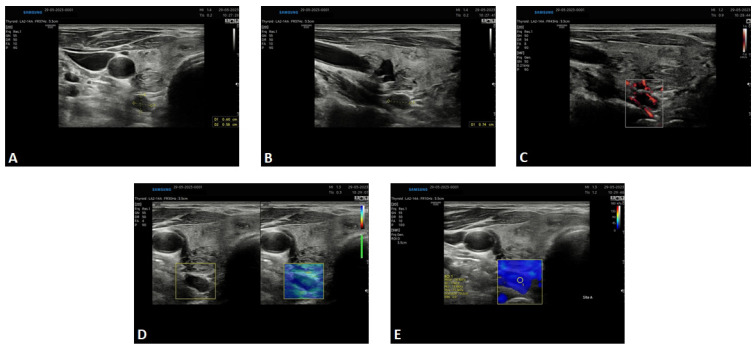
Ultrasound picture of the lesion corresponding to the parathyroid adenoma. The USG was performed March 2023. (A)—conventional ultrasound examination; transverse section of the neck; hypoechogenic oval lesion on the postero-lateral wall of the thyroid, located extracapsularly. (B)—conventional ultrasound examination; a longitudinal section of the neck; hypoechogenic oval lesion on the posteriori wall of the thyroid, located extracapsularly. (C)—the polar vessel sign on superb microvascular imaging. (D)—high elasticity of the lesion depicted by strain elastography. (E)—high elasticity of the lesion depicted by shear wave elastography. All features suggestive of parathyroid adenoma.
